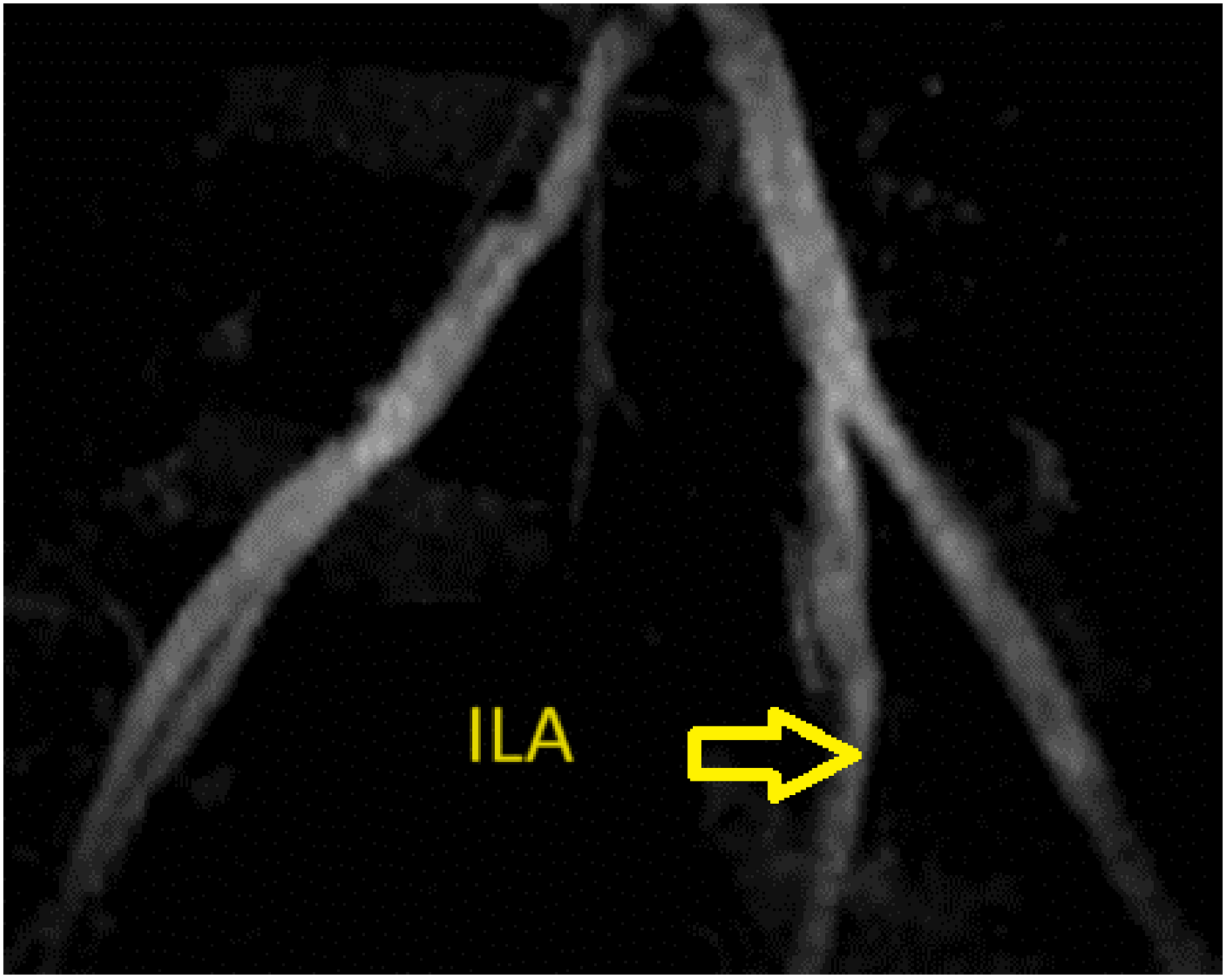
Representative images of the allograft obtained by preoperative enhanced computed tomography. There was mild stenosis of the left internal iliac artery.
